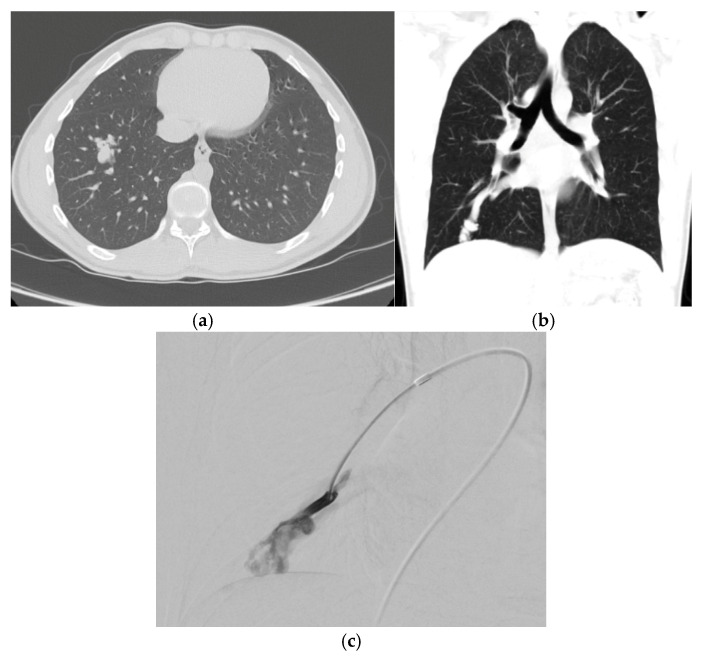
(a) Non-contrast axial computed tomography (CT) scan demonstrates a right lower lobe pulmonary arteriovenous malformation (PAVM) with clearly defined vessels but some residual surrounding ground glass in an adolescent patient with history of hypoxemia and transient ischemic attack. (b) Coronal non-contrast CT again demonstrating the large draining pulmonary vein with developing nidus. (c) Corresponding selective pulmonary angiogram demonstrates the developing nidus.
